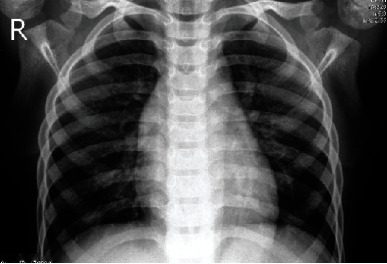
Pneumonia-affected chest X-ray.
